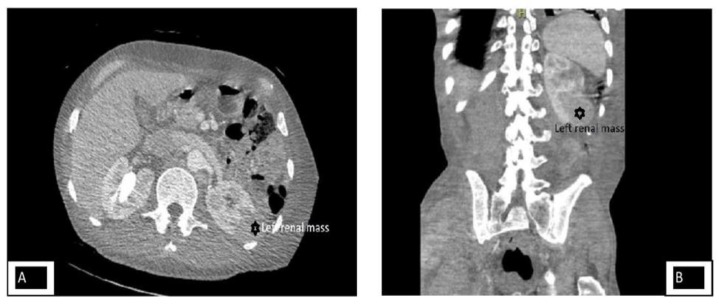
Panel A: Cross-section of CT abdomen and pelvis showing left renal mass. Panel B: Coronal section of CT abdomen and pelvis showing left renal mass
